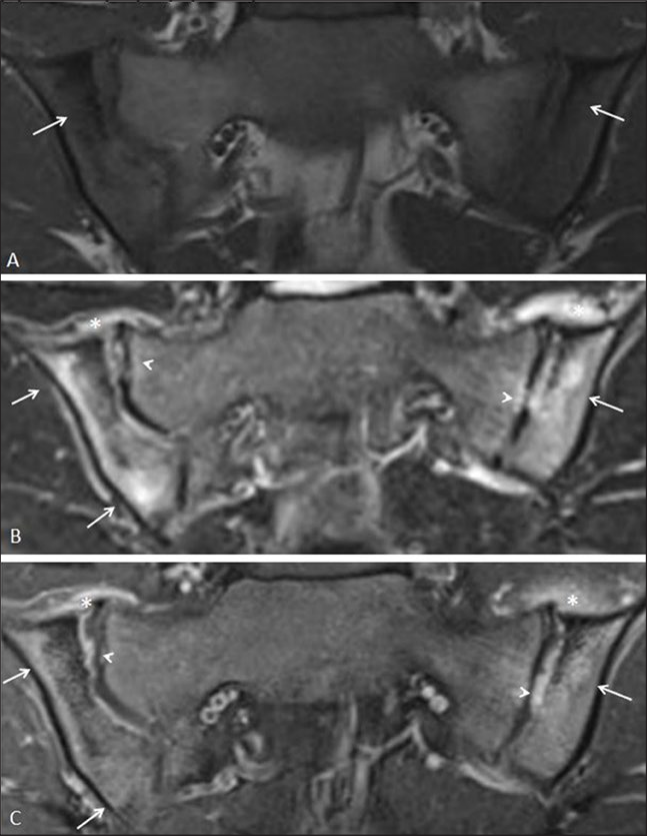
Multiple active and structural features of sacroiliitis seen on MRI in a 16-year-old girl with JSpA. a. Semicoronal T1-weighted image shows erosions and sclerosis in both sacroiliacal joints (arrows). b. Semicoronal STIR image shows high signal in the joint space of both sacroiliacal joints (short arrows) and bone marrow edema at the left iliac side (arrows). There is extensive capsulitis on both sides (asterisk). c. Corresponding contrast-enhanced fat-saturated T1-weighted image shows synovial enhancement in the erosions (short arrows) and enhancement at the site of the edema (arrows). Note also the enhancing capsulitis on both sides (asterisk).
